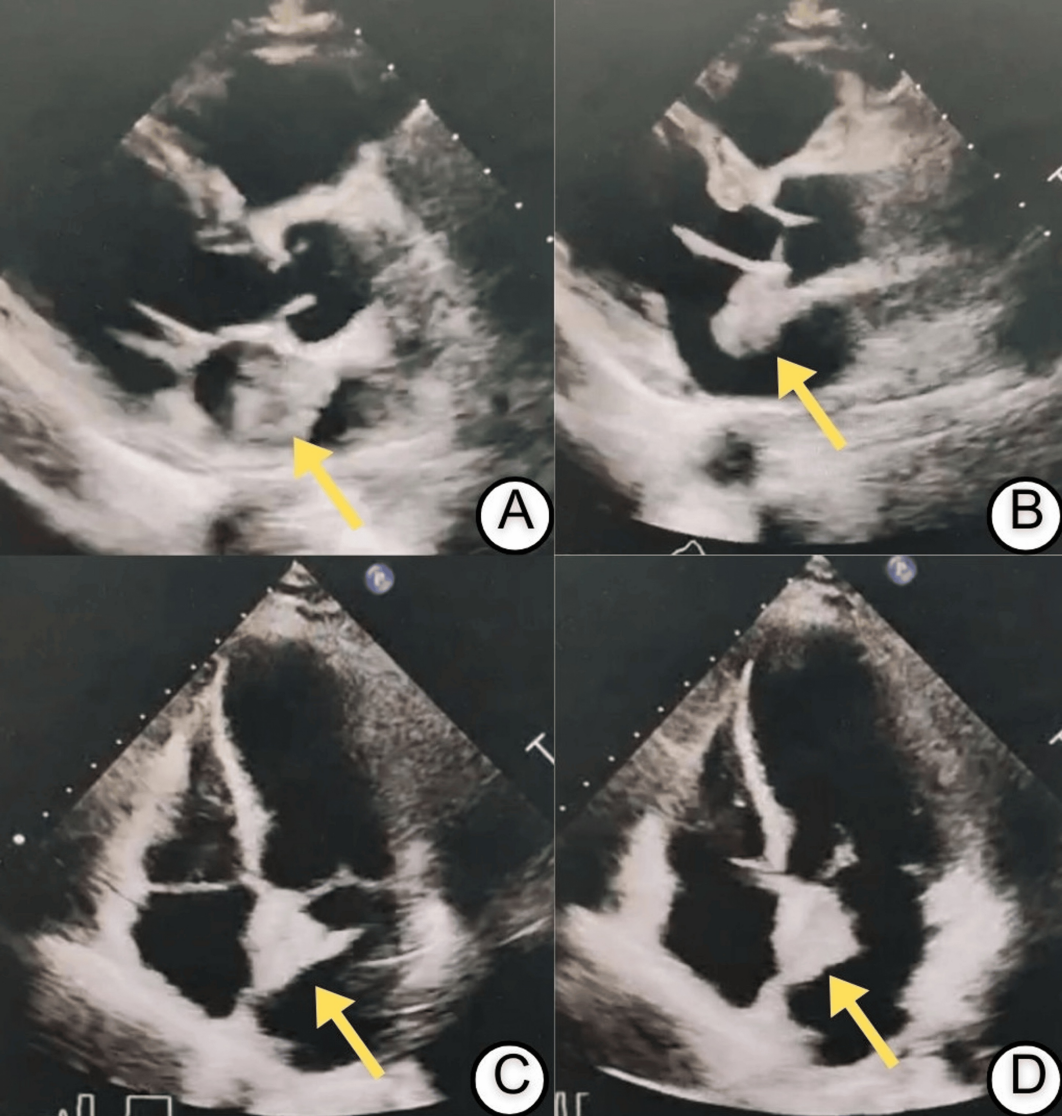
Transthoracic echocardiography demonstrating a left atrial myxoma. (A, B) Parasternal long-axis views showing a large, pedunculated, and mobile mass (yellow arrows), measuring approximately 2.6 × 3.2 cm, attached to the interatrial septum and prolapsing across the mitral valve into the left ventricle during diastole. (C, D) Apical four-chamber views confirming the left atrial mass with dynamic prolapse into the left ventricle, consistent with a left atrial myxoma.
